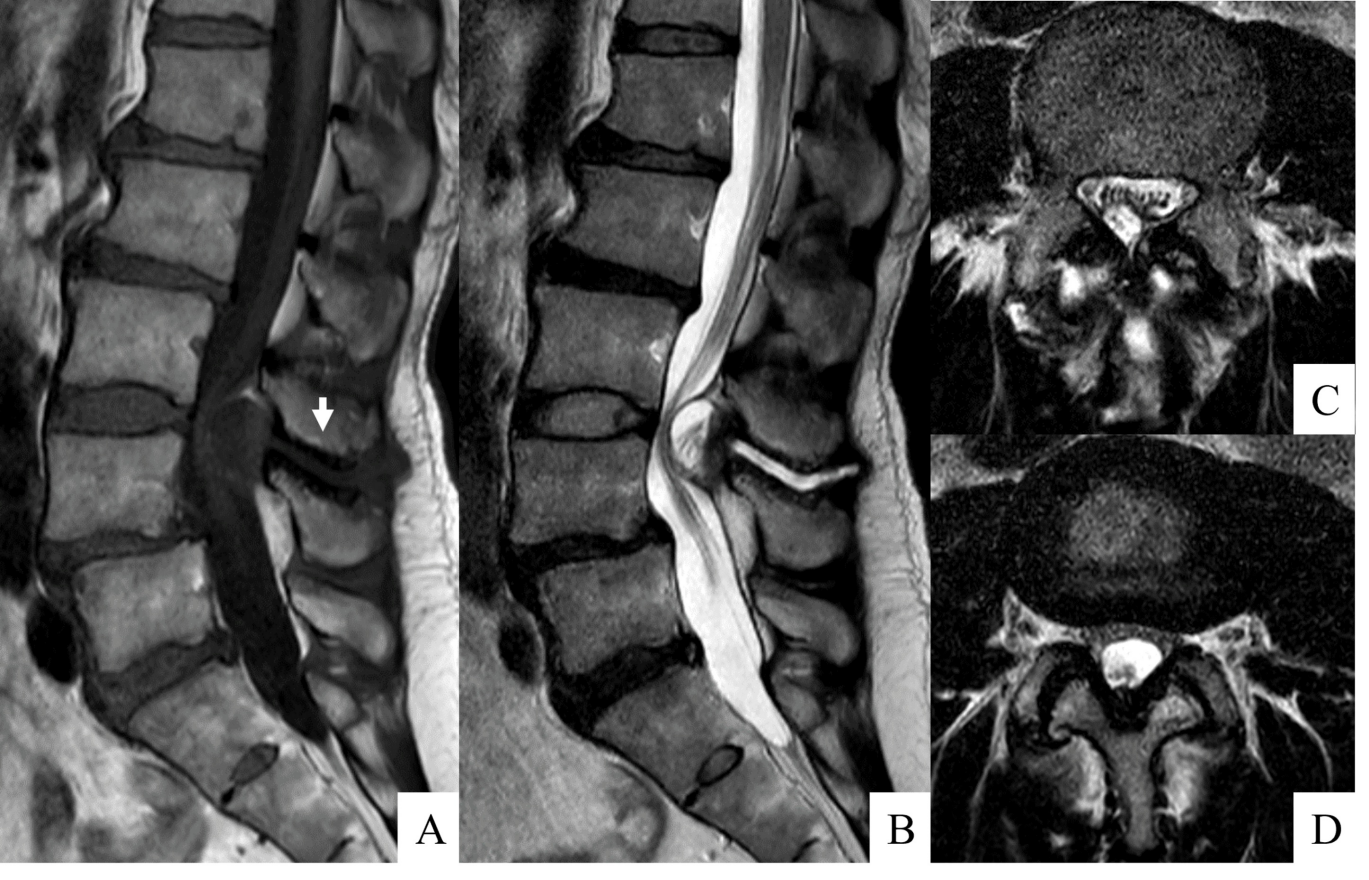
Preoperative magnetic resonance imaging Magnetic resonance imaging on T1 sagittal view shows the presence of a low intensity area (A; white arrow). Those on T2 sagittal and axial views show a high intensity area (B, C) within the L3-L4 interspinous space, accompanied by the development of an intraspinal cyst. The T2 axial view at L3-L4 disc level shows a central canal stenosis due to the cyst (D).
